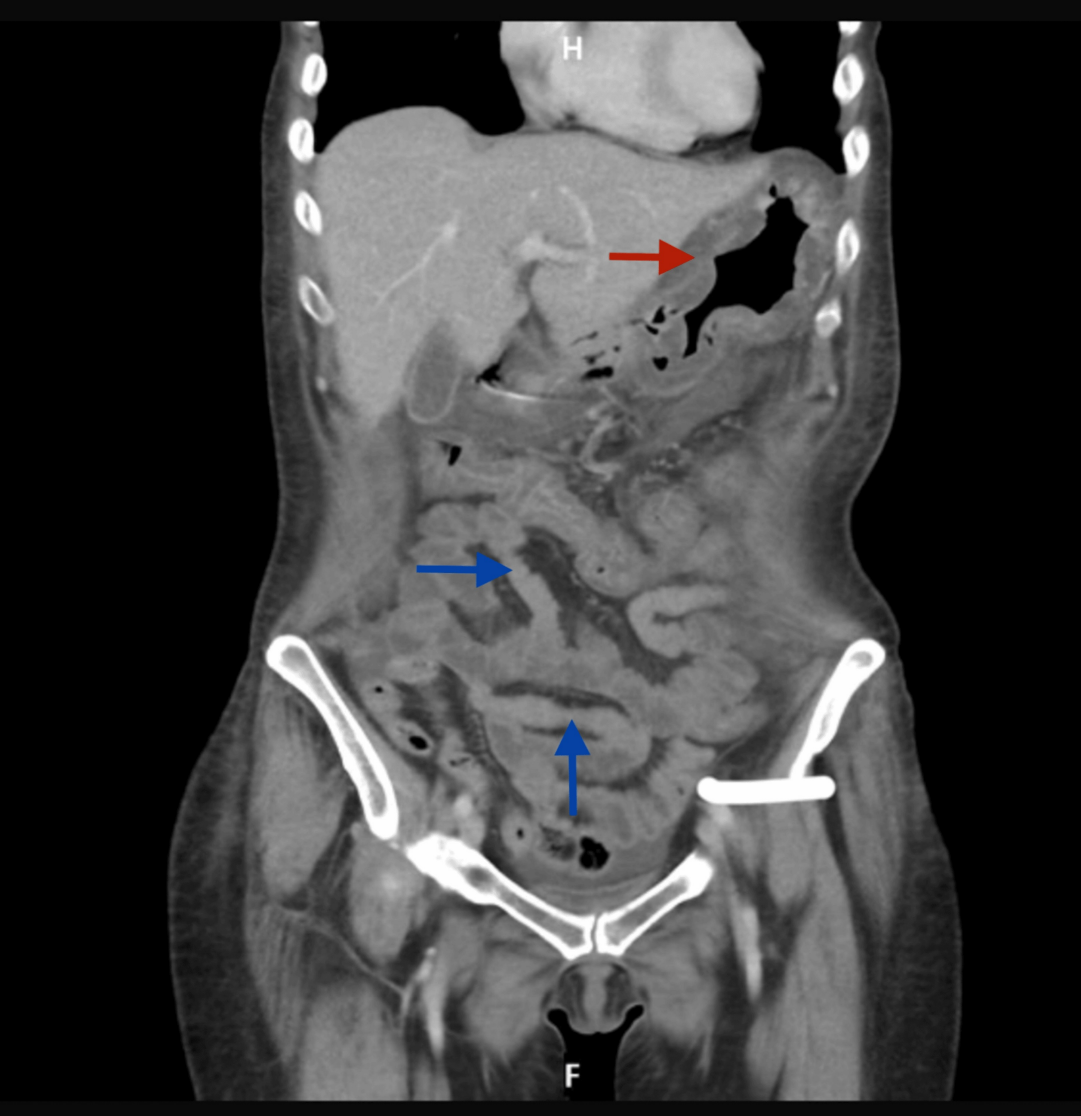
CT abdomen pelvis. The blue arrow points towards small bowel loops filled with fluids with intervening segment of collapsed bowel. The red arrow points towards diffuse gastric wall oedema, upper abdominal fat stranding, and mild ascites
